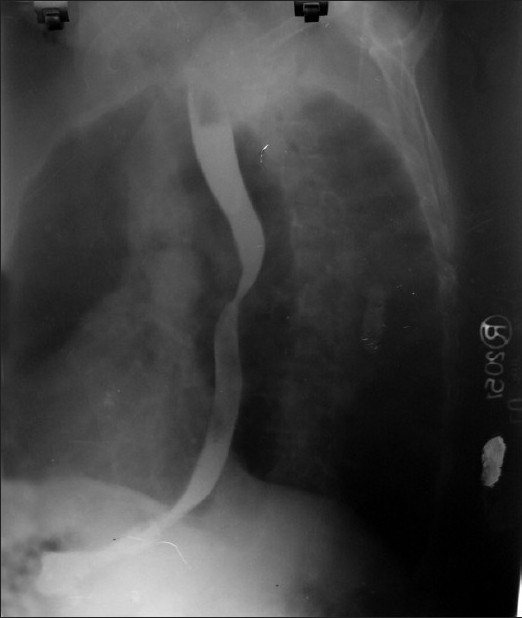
Radiograph of barium study showing anterior indentation of oesophagus
